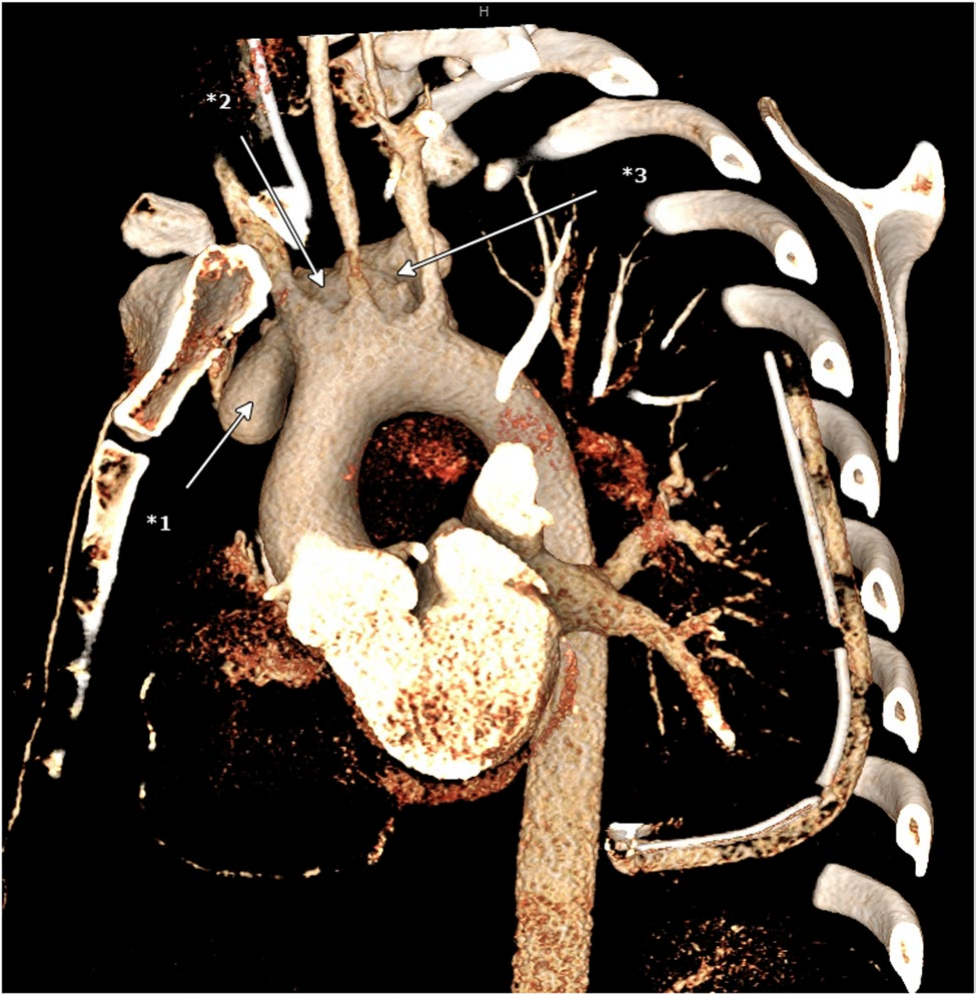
3D reconstruction of the CT scan, *1-*3 pseudoaneurysm
